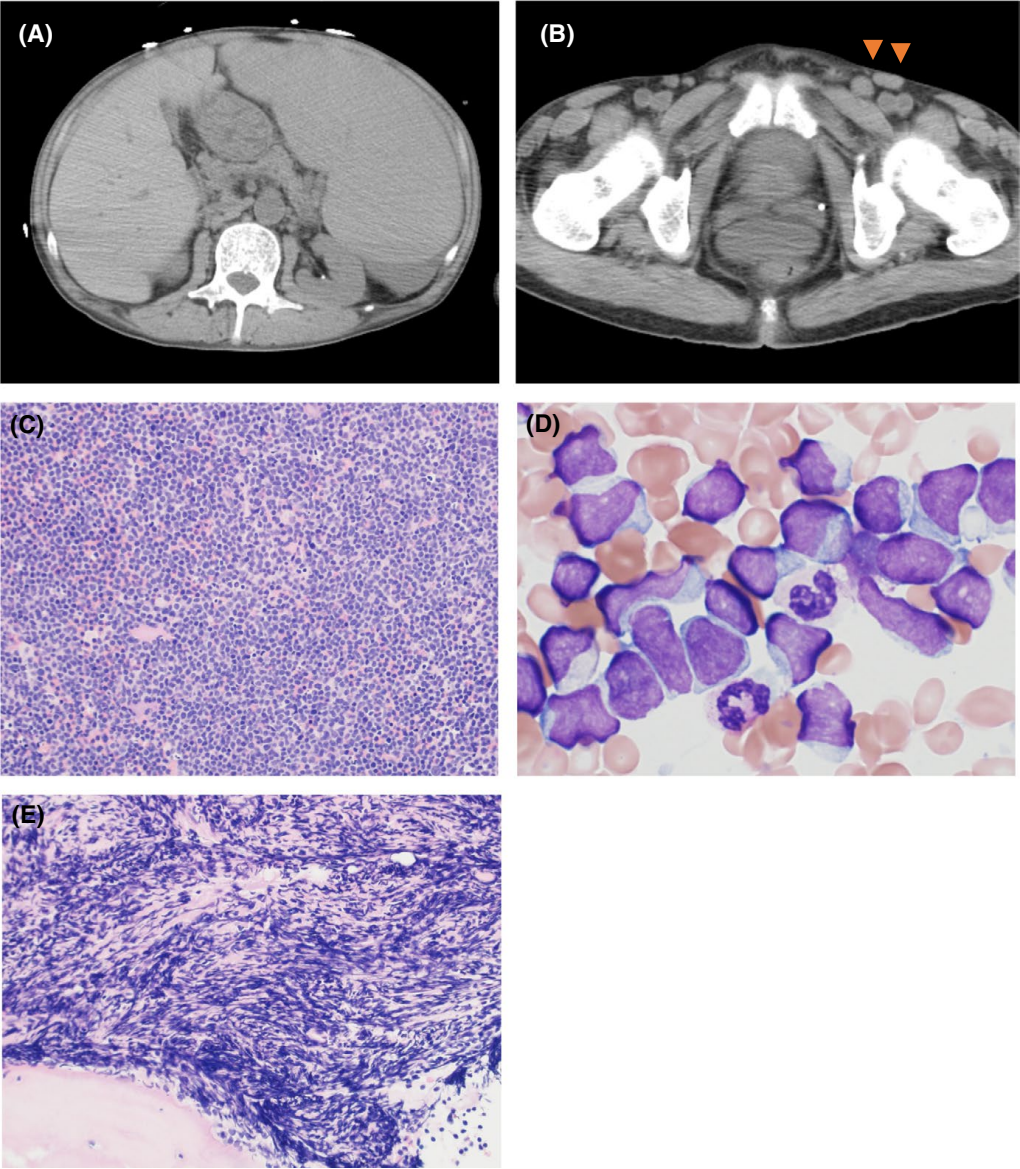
Computed tomography revealed splenomegaly (A) and inguinal lymphadenopathy (B). Markedly hypercellular marrow was occupied by blasts (C), presenting agranular small cytoplasm and fine chromatin (D). Bone marrow biopsy showed severe fibrosis (MF‐3) (E) (magnification of all images ×400).
